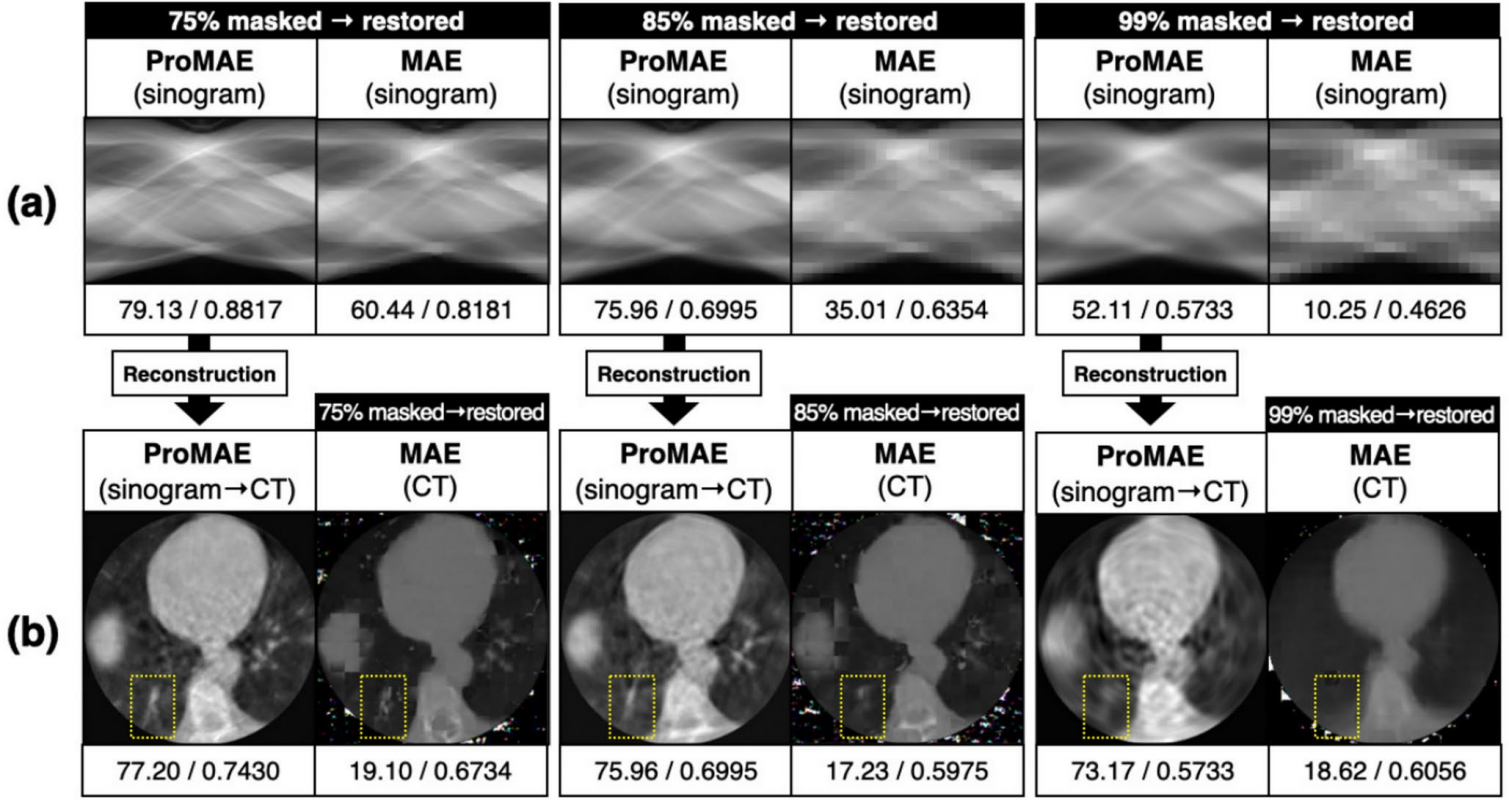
Pre-training Results: a A comparison of the restoration performance of masked sinograms between ProMAE and MAE. b A comparison of the CT images reconstructed from the sinogram restored by ProMAE with those restored by MAE. The yellow box indicates the lesion location, and the numbers at the bottom of each restored image represent the PSNR (left) and SSIM (right) of the restored image relative to the original image
